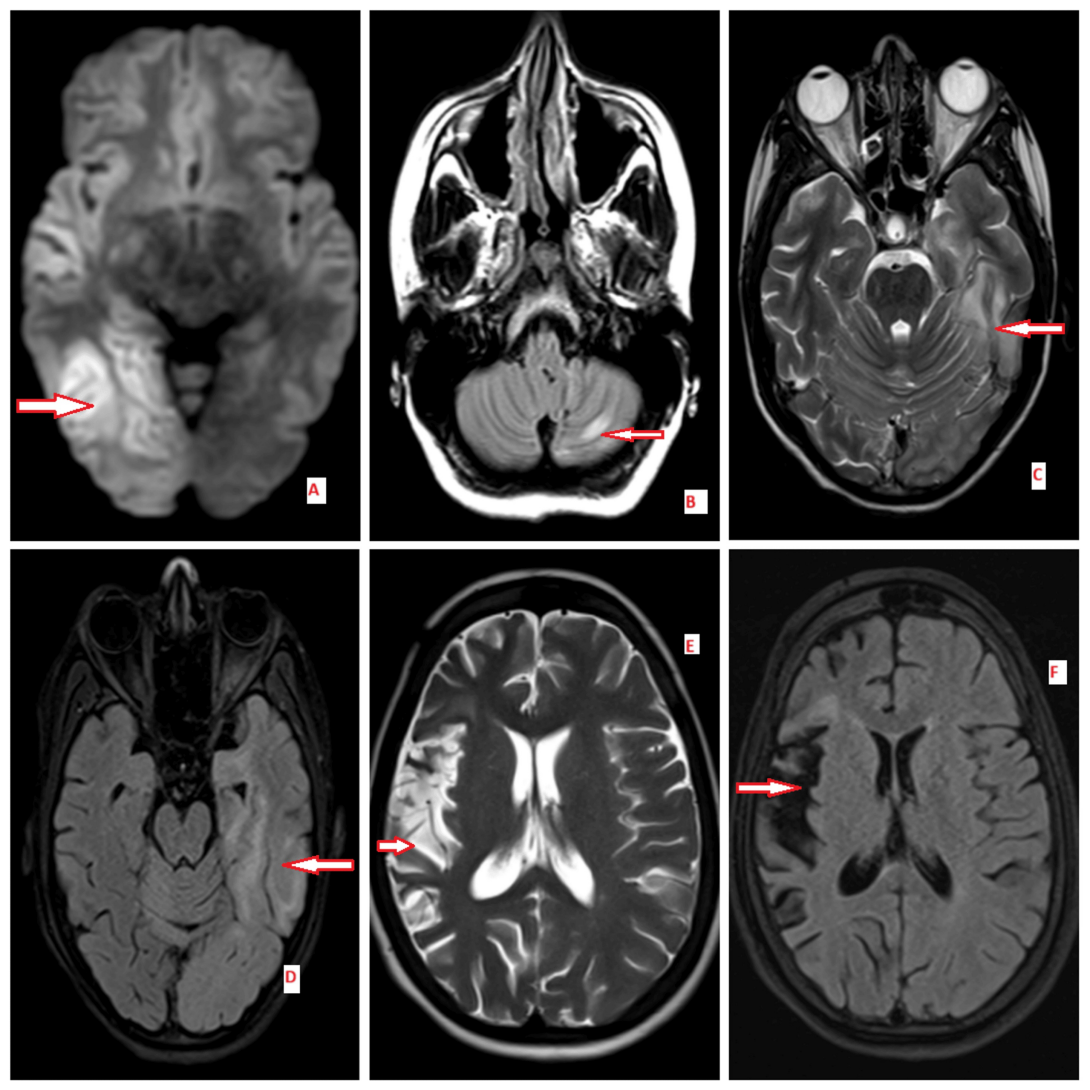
A and B MRI images (Case 2): T2/FLAIR hyperintensities in the right > left temporal and hippocampus. C and D MRI images (Case 3): T2/FLAIR hyperintensities with diffusion restriction in the left temporal and occipital area. E and F MRI images (Case 4): T2/FLAIR right hippocampus and cerebral hemisphere atrophy suggestive of Rasmussen's encephalitis. Red arrow: lesion MRI: magnetic resonance imaging, FLAIR: fluid-attenuated inversion recovery
